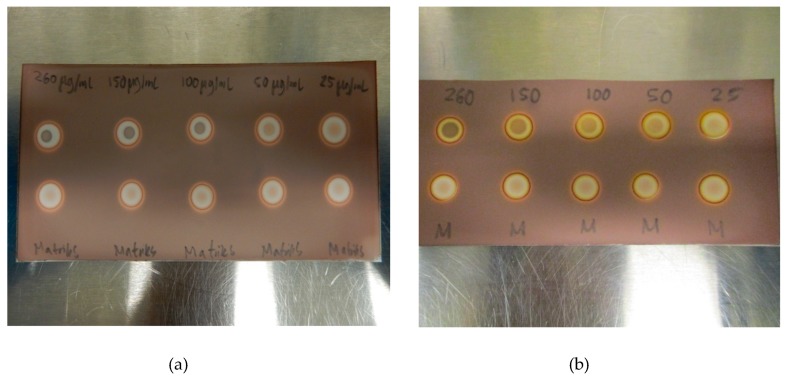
(a) LoD determination for TBA in the [^18^F]PSMA-1007 matrix without antioxidant and. (b) LoD determination for TBA in the [^18^F]PSMA-1007 matrix with antioxidant.
